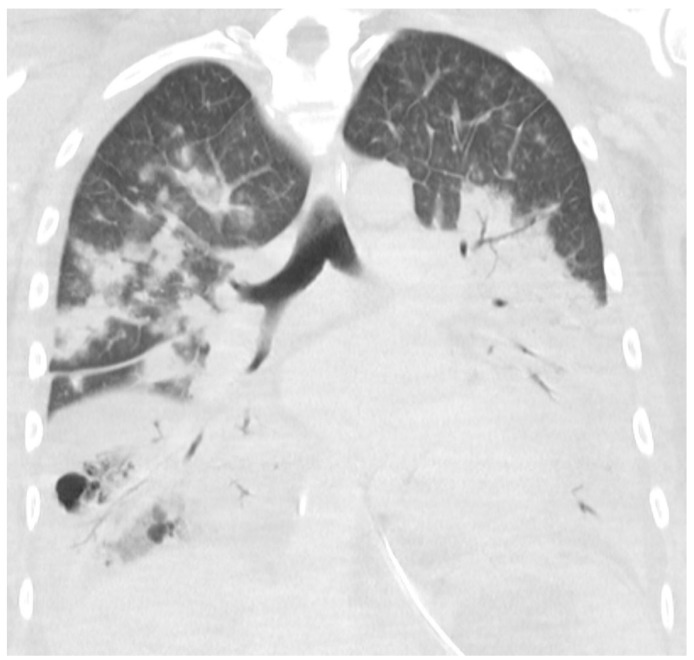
CT scan of the chest with coronal reconstruction. Massive bilateral consolidation with air bronchogram and minimal pleural effusion at day 4.
